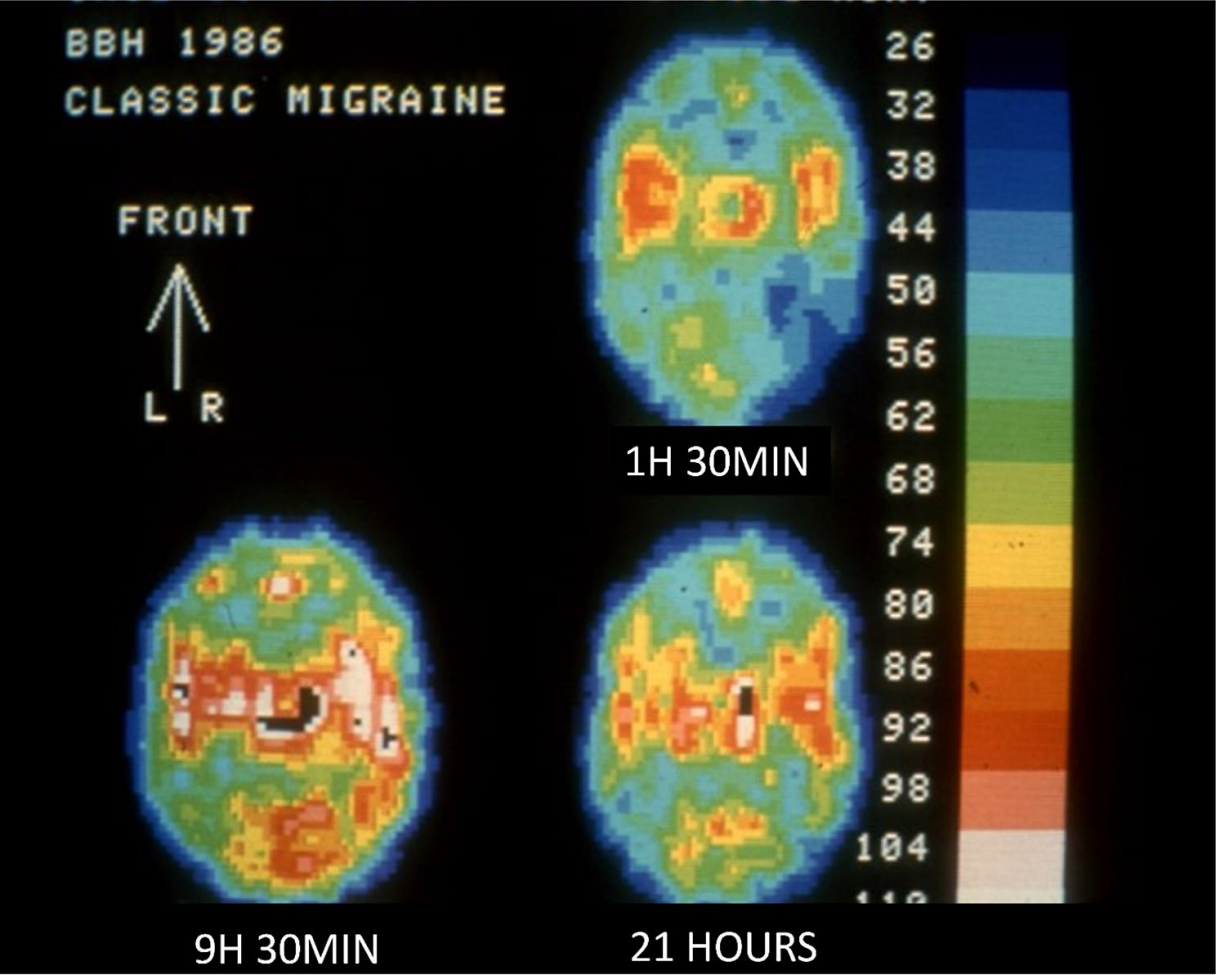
SPECT study of spontaneous attack of migraine with aura. The top picture shows the initial low flow (blue colour) in the right occipital-parietal area. Below to the left the rCBF has changed into hyperperfusion (red and yellow colour). Finally rCBF is normal and symmetrical in the right sided picture. Modified from [21]
